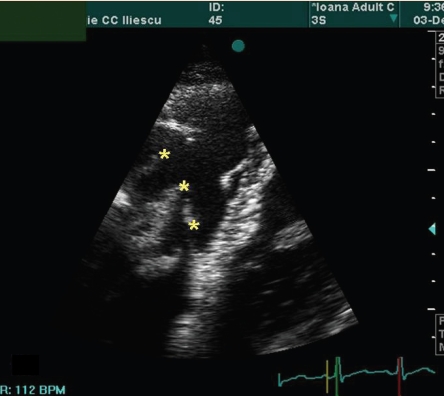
Apical four–chamber view. Diastole. Zoom of the masses (asterix) inside the right ventricular inflow, just under the tricuspid annular plane.
